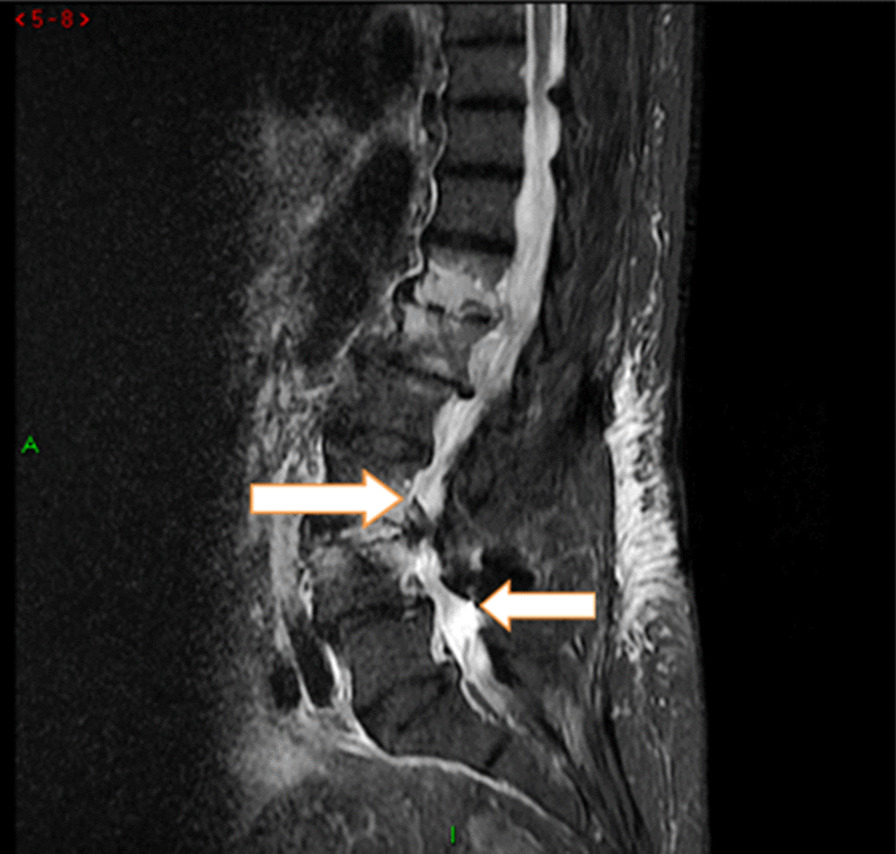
Sagittal stir magnetic resonance imaging showing an abnormal T12–L1 and L3–L4 vertebral body signal with epidural thickness (arrows)
